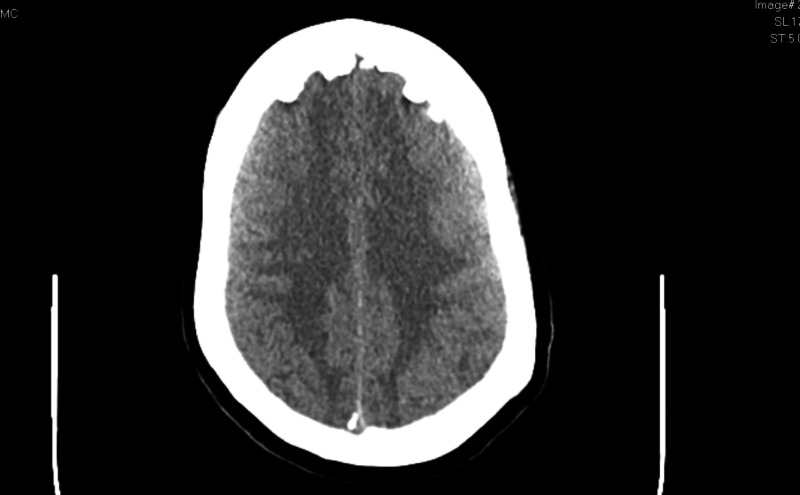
Case 1 head CT on admission April 11, 2020
